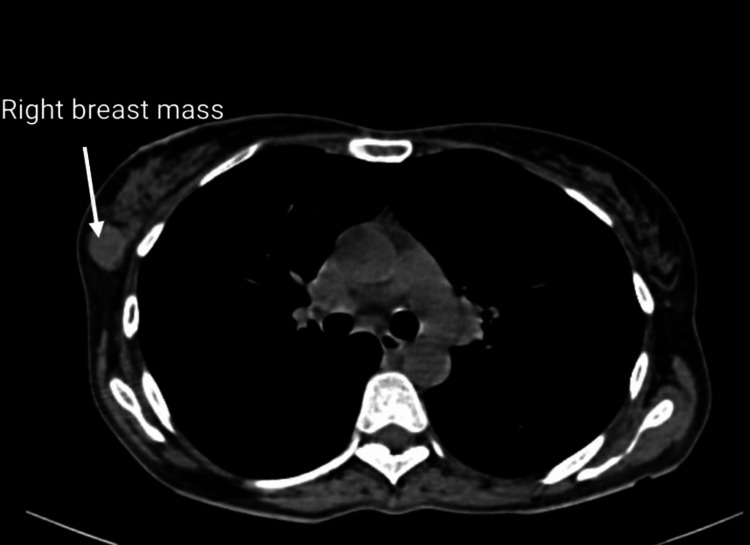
Sagittal view of the computed tomography of the thorax showing a tumor (arrow) in the upper outer quadrant of the right breast
